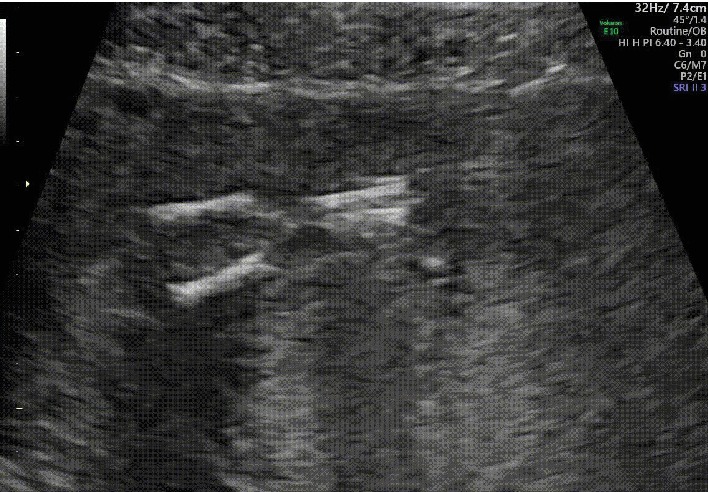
Gray-scale ultrasonography at the 14 weeks of gestation. Two femurs, two tibiae, and two fibulae were visualized.
